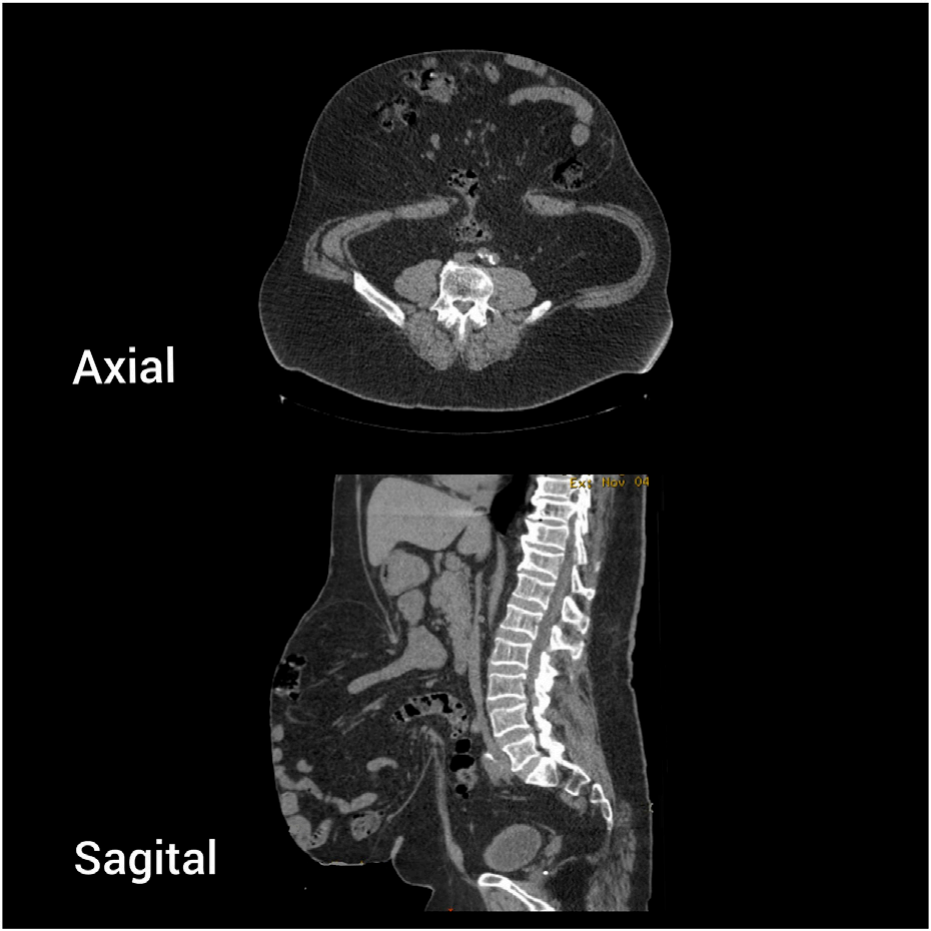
Case 2 - Preoperative CT scan. Axial and sagittal view.
